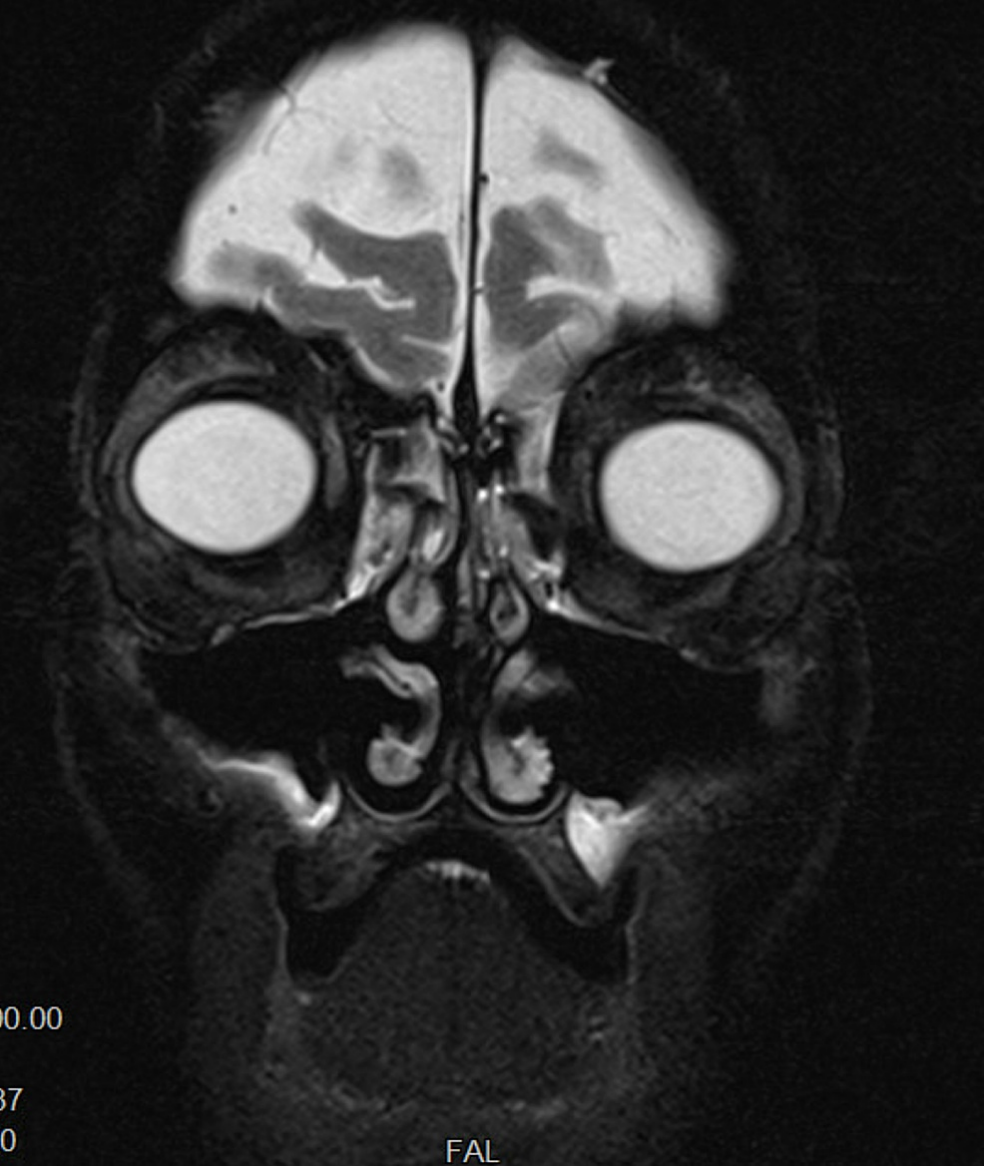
Coronal MRI view of the orbits.
